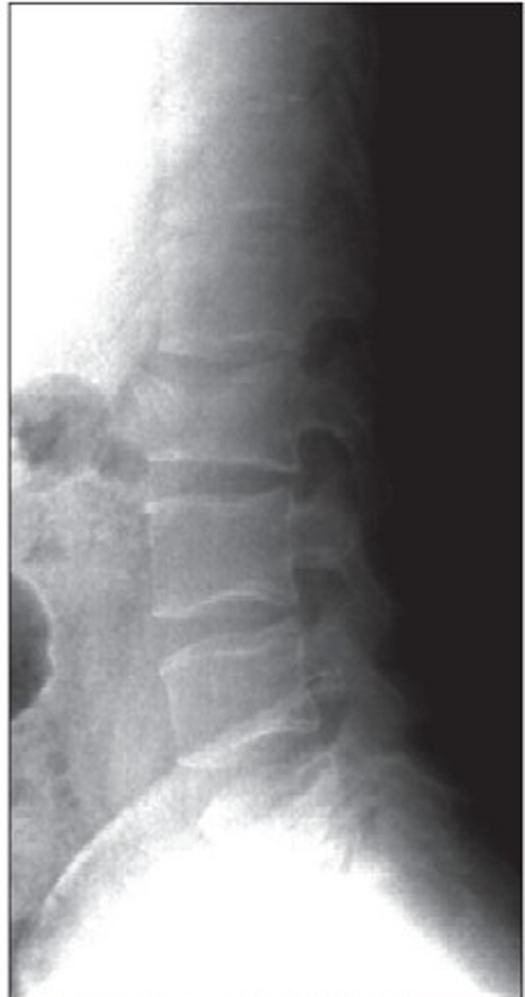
Preoperative X-ray of L1 fracture, B type with LSC 7
